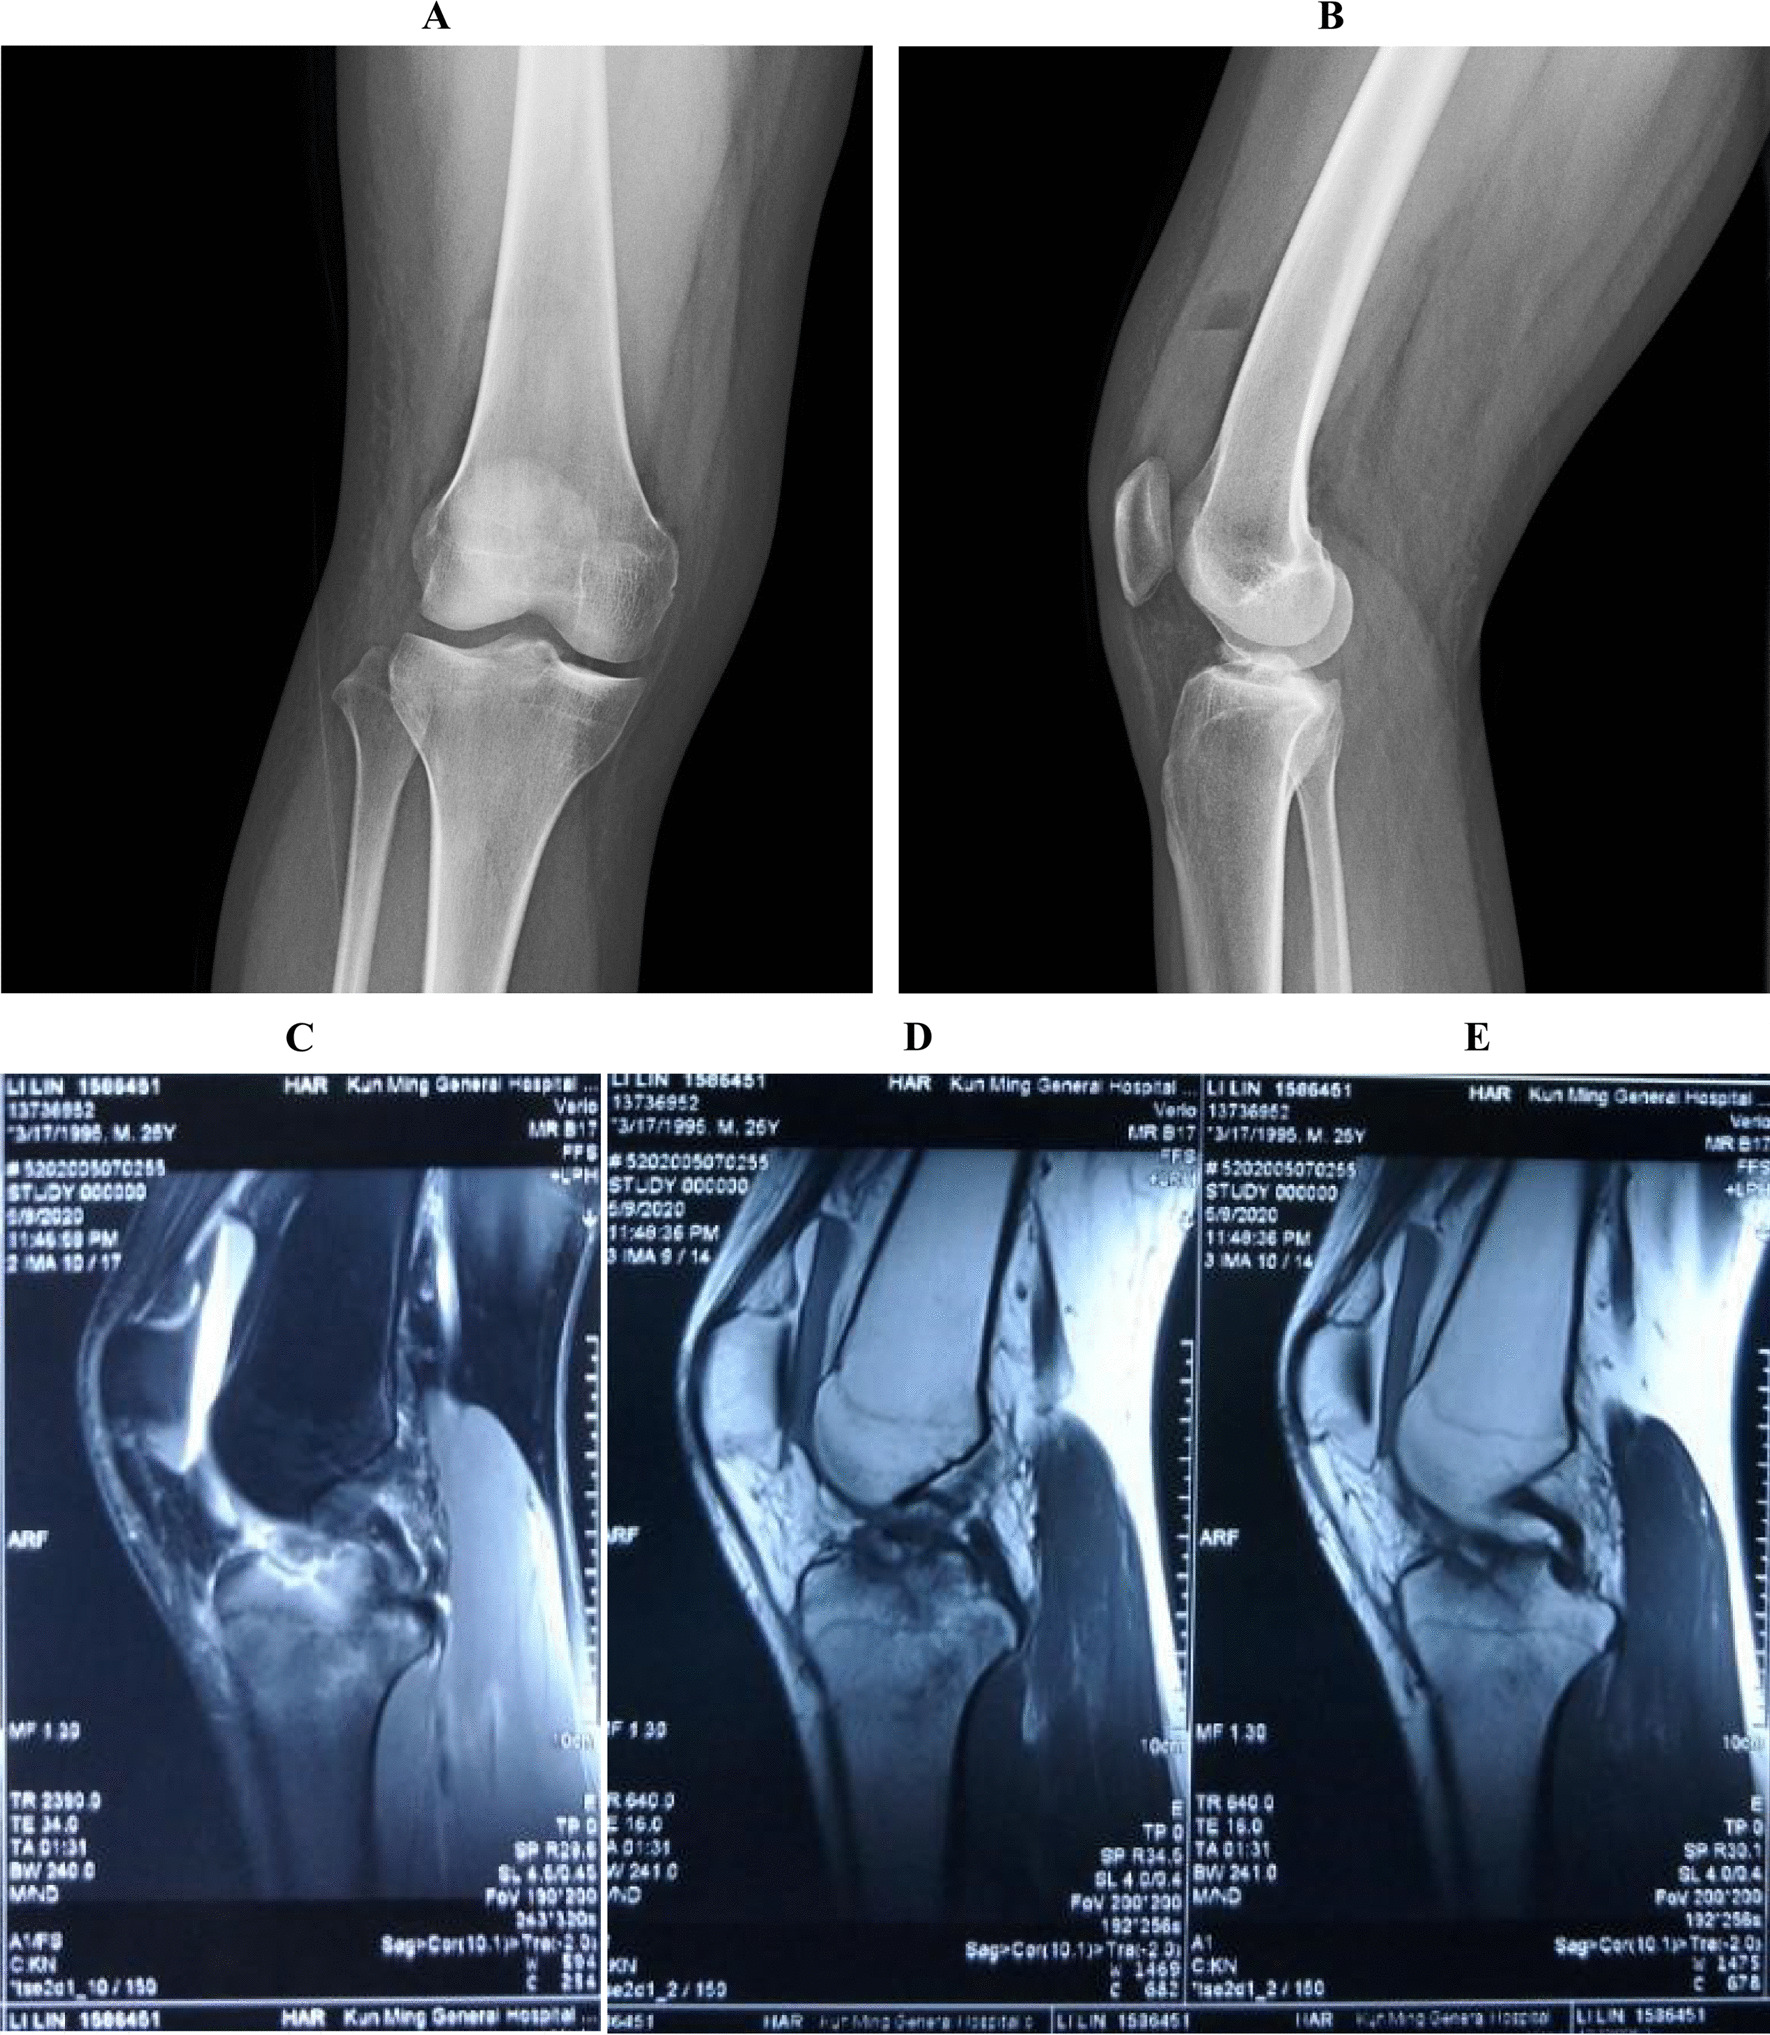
Preoperative detection results of cases. A, B X-ray detection of preoperative intercondylar ridge fracture in patients; C–E magnetic resonance imaging detection of intercondylar crest fracture before operation
